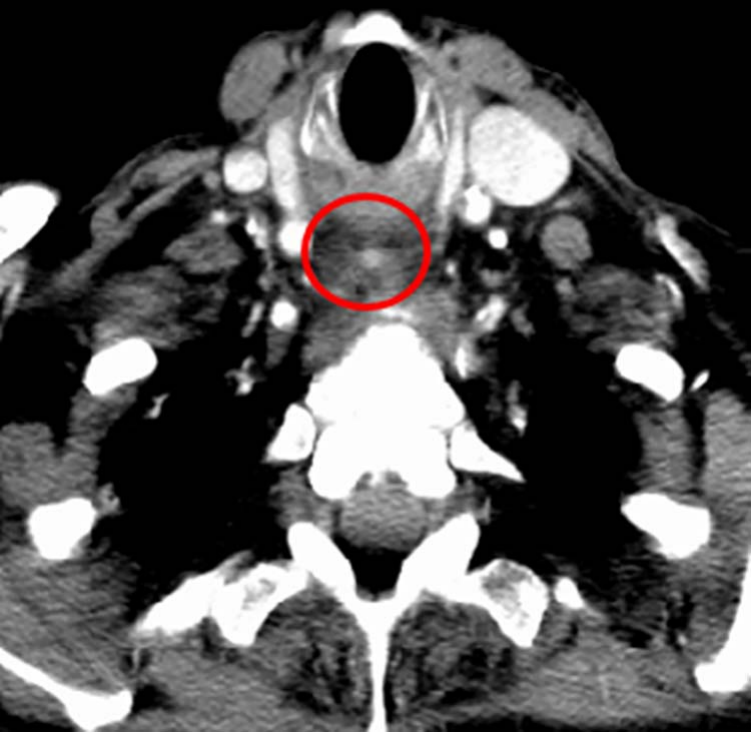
CT neck; post-cricoid mass containing an air locule with a 3-mm hyperdense mass (red circle) in the caudal end of this.
